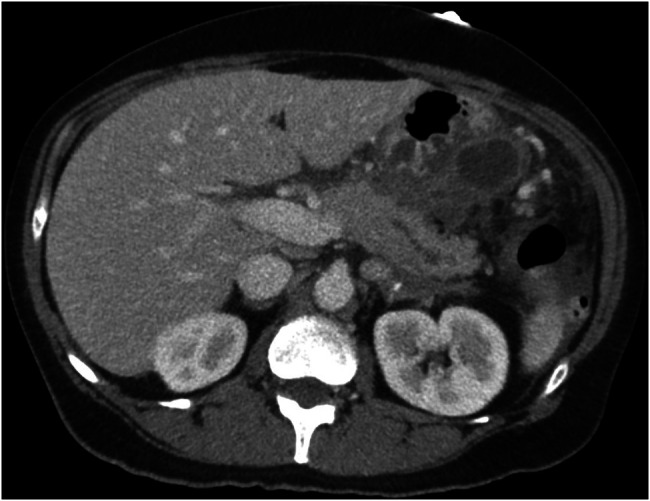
Computed tomography scan on index presentation consistent with peripancreatic fluid collection, dilated pancreatic duct, and changes consistent with pancreatitis.
